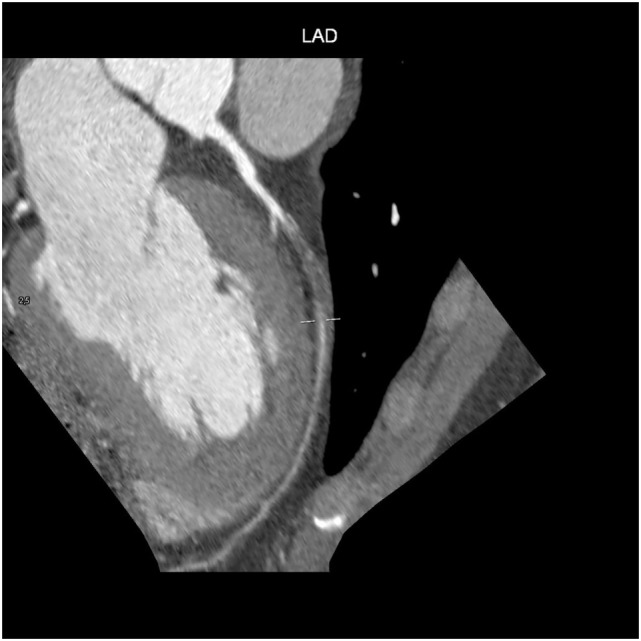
Coronary CT: chronic total occlusion of left anterior descending artery.
